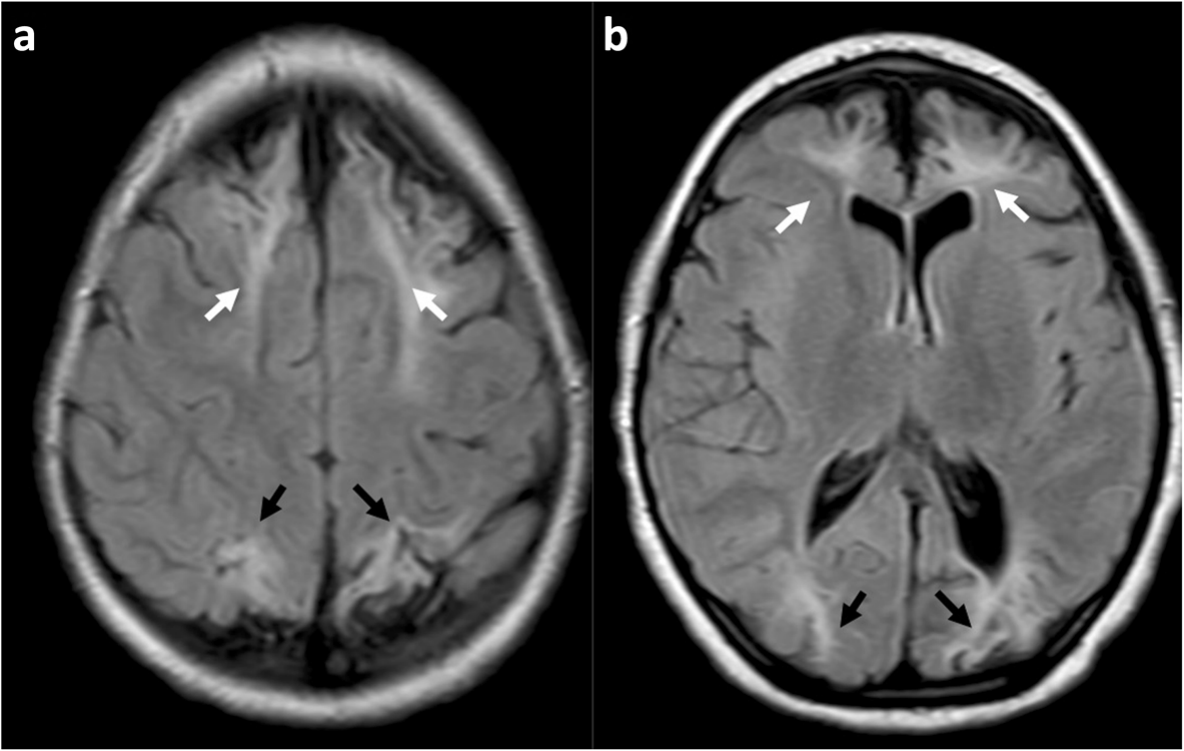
a, b Sequential axial FLAIR images in a 4-year and 9-month-old girl, who sustained a partial-prolonged hypoxic-ischaemic injury at term gestation during prolonged labour, demonstrating bilateral, symmetric volume loss with abnormal underlying white matter high signal in the anterior watershed (white arrows), apparently discontinuous from similar involvement of the posterior watershed (black arrows). This is perceived as a classical watershed distribution but does not demonstrate the watershed continuum in its entirety
